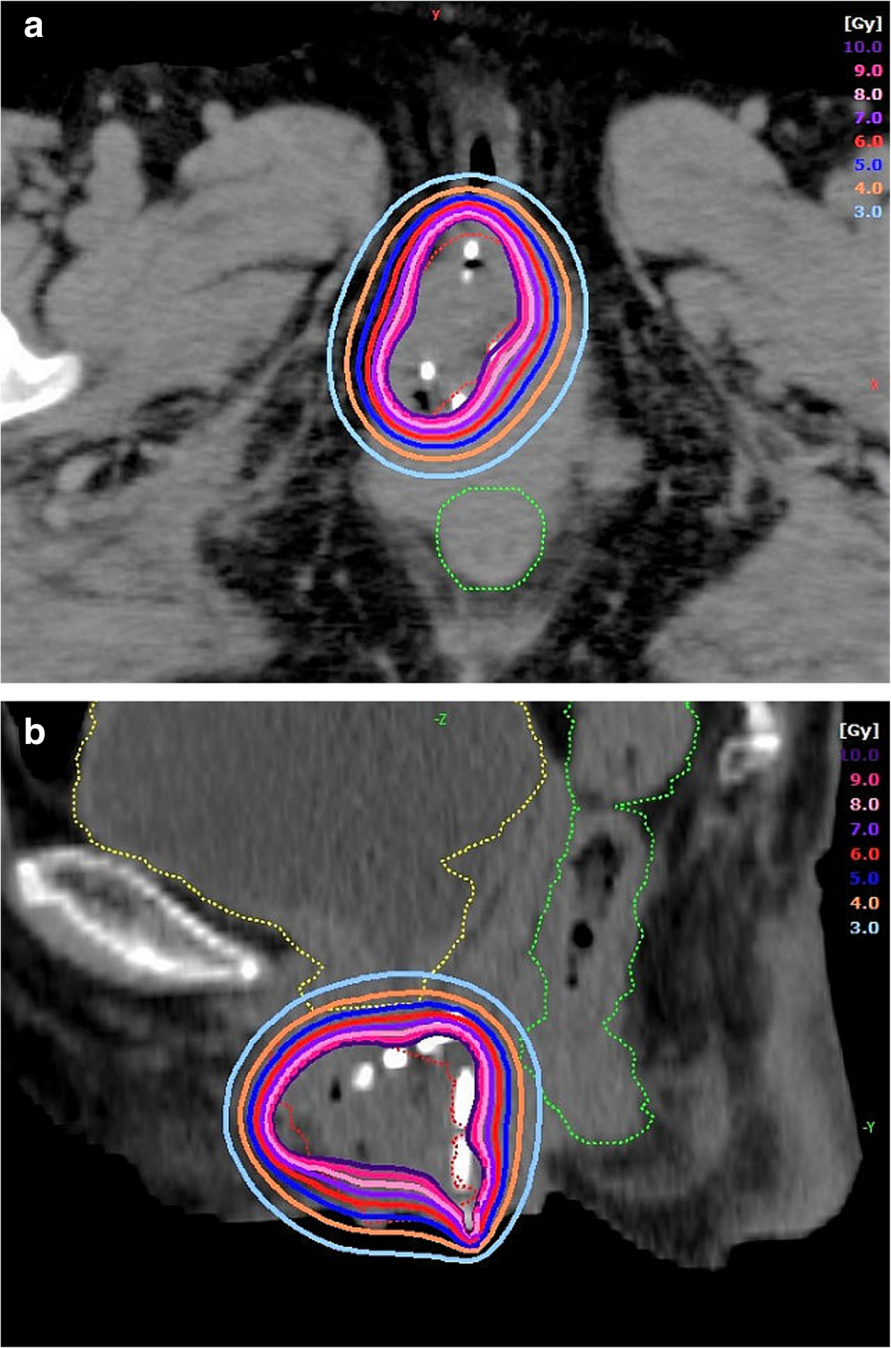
a Axial and b sagittal computed tomography scans with high-dose-rate interstitial brachytherapy dosimetry from 10- to 3-Gy isodose lines. The the gross tumor volume (red dotted line) was well covered by the prescribed 6-Gy isodose line. The rectum (green dotted line) and bladder (yellow dotted line) are only slightly irradiated
